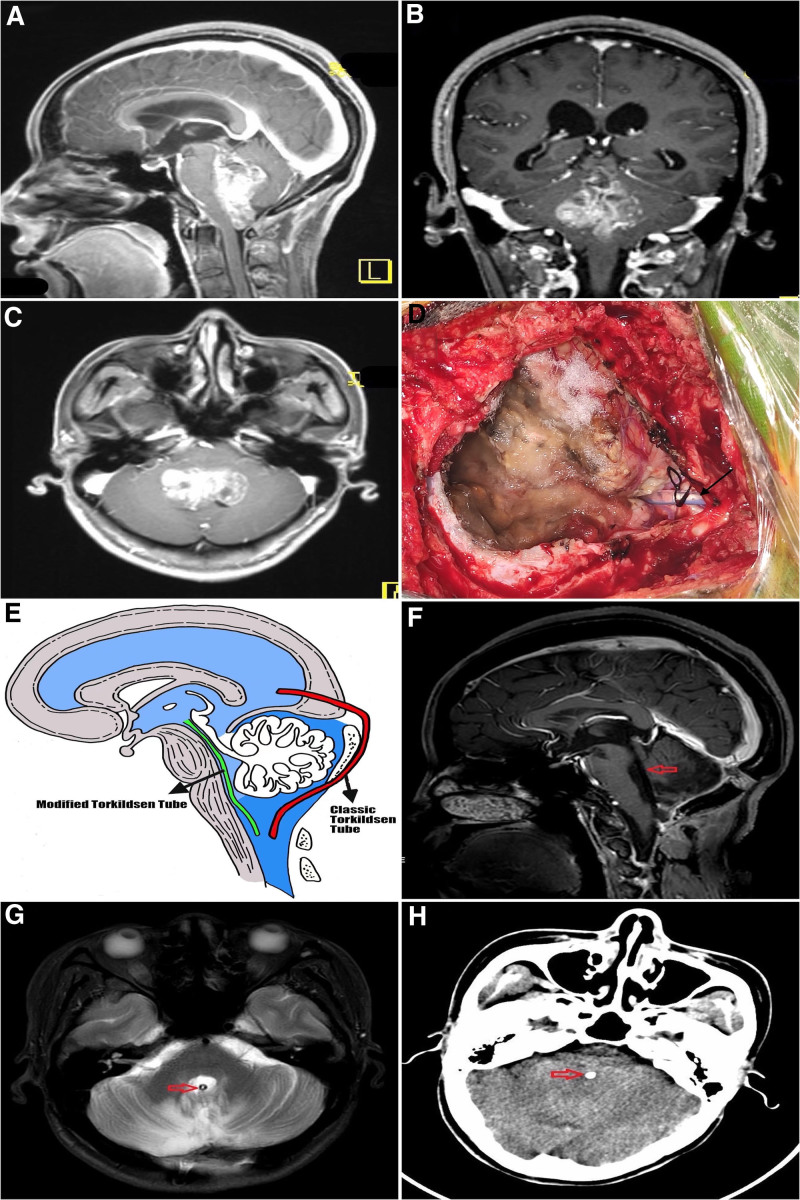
Are pre-, intra- and postoperative radiological and surgical images one of the patients who received AFVCM shunting. (A) Is preoperative sagittal MRI showing fourth ventricular tumor. (B) Is preoperative coronal MRI showing forth ventricular tumor. (C) Is preoperative axial MRI showing forth ventricular tumor. (D) Is an intraoperative image showing the AFVCM shunt in situ with a non-absorbable suture fixing the catheter onto the dura on the foramen magna (black arrow = catheter + suture). (E) Is sketched image showing the precise location of the AFVCM shunt/tube as against the classic Torkildsen shunt/tube. (F) Is postoperative sagittal MRI showing the fourth ventricular after tumor resection (red arrow = the fourth ventricle). (G) Is postoperative axial MRI showing the AFVCM shut in situ after tumor resection (red arrow = AFVCM shunt). (H) Is postoperative CT scan showing the modified Torkildsen shut in situ after tumor resection (red arrow = AFVCM shunt). AFVCM = aqueduct-fourth ventricle-cisterna magna, MRI = magnetic resonance imaging.
